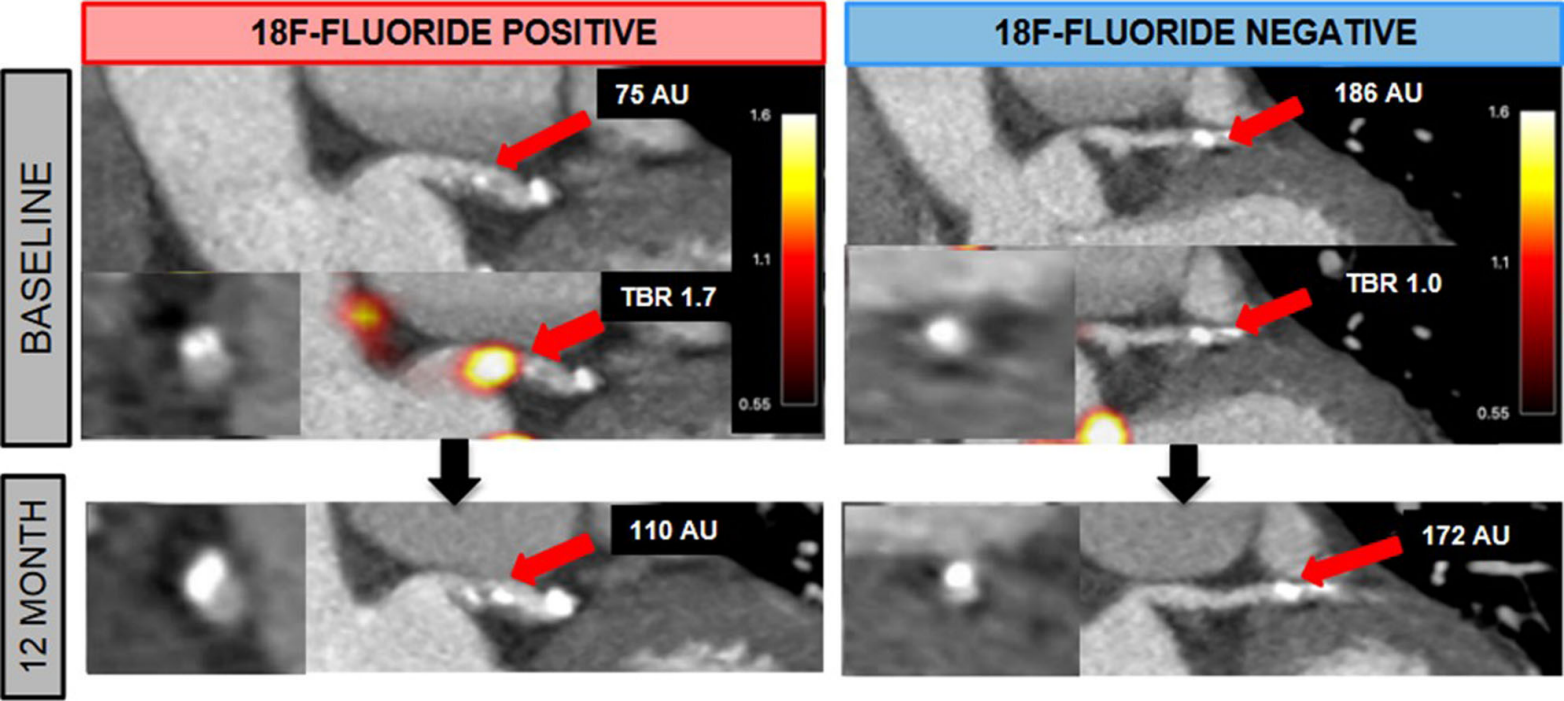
^18^F-sodium fluoride positron emission tomography to predict progression of coronary calcification. Examples of two patients undergoing ^18^F-sodium fluoride (^18^F-NaF) positron emission tomography (PET) and coronary computed tomography angiography at baseline and follow-up. The patient showing uptake of ^18^F-NaF (18F-fluoride positive) had progression of coronary calcification at follow-up whereas the patient without uptake (18F-fluoride negative) did not show changes. Reproduced with permission from Doris et al^12^
